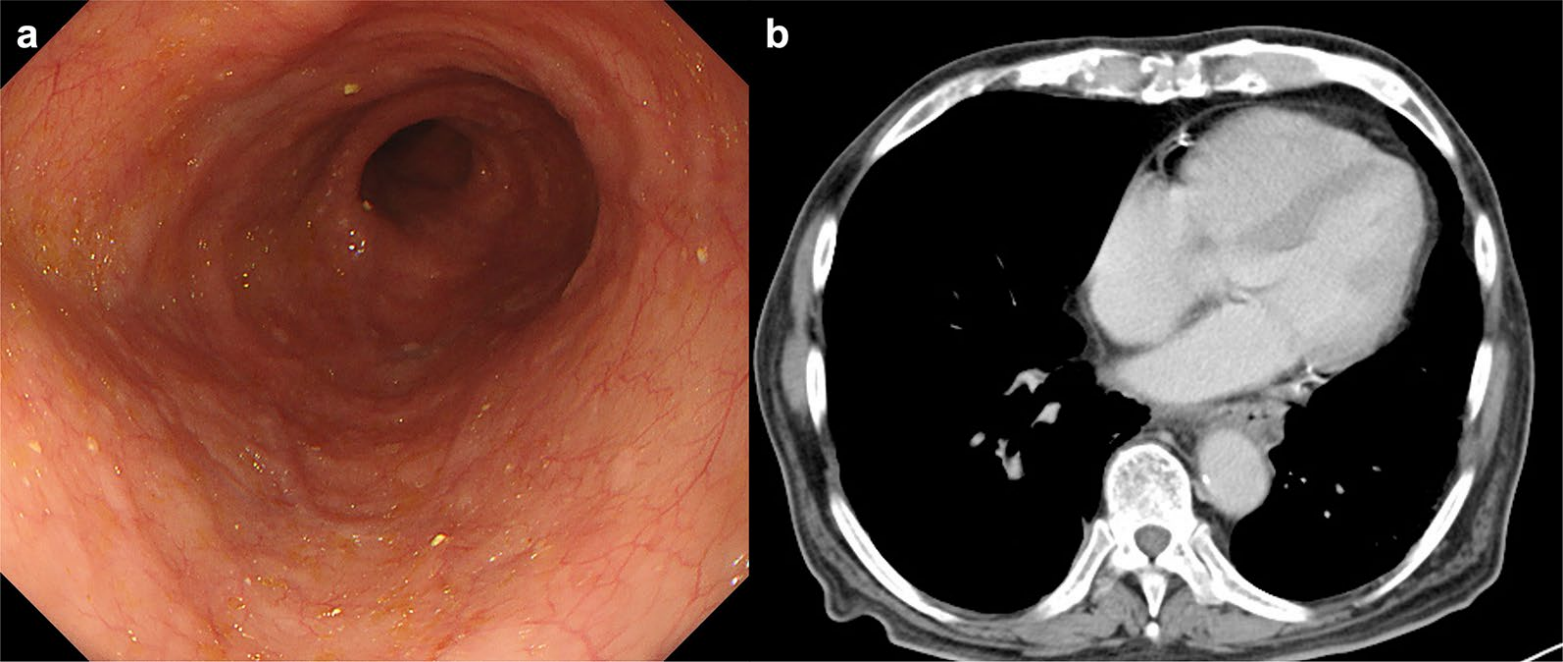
Postoperative findings. a Upper endoscopy image of the lower-thoracic esophagus taken 2 months post-surgery showing no sign of esophageal stricture or malignant mucosal invasion. b Contrast enhanced computed tomography of the chest taken 10 months post-surgery showing no sign of disease progression
